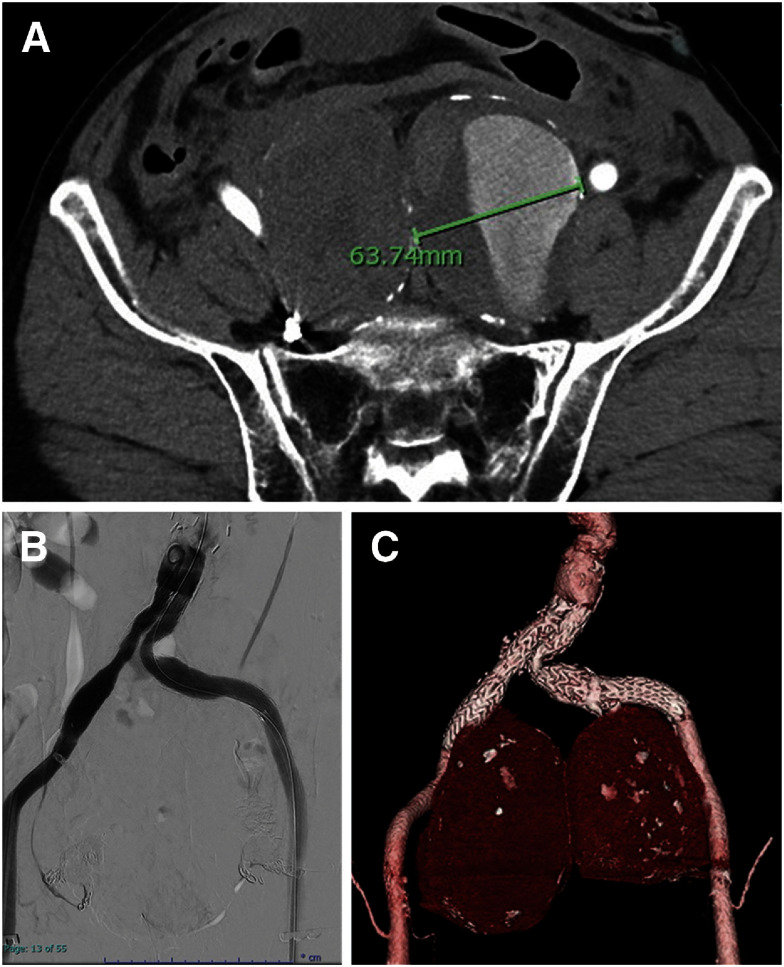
Fig. 1 (A) Preoperative computed tomography image. (B) Intraoperative final angiographic portrait. (C) Postoperative computed tomography image (3D reconstruction). 3D: three-dimensional
